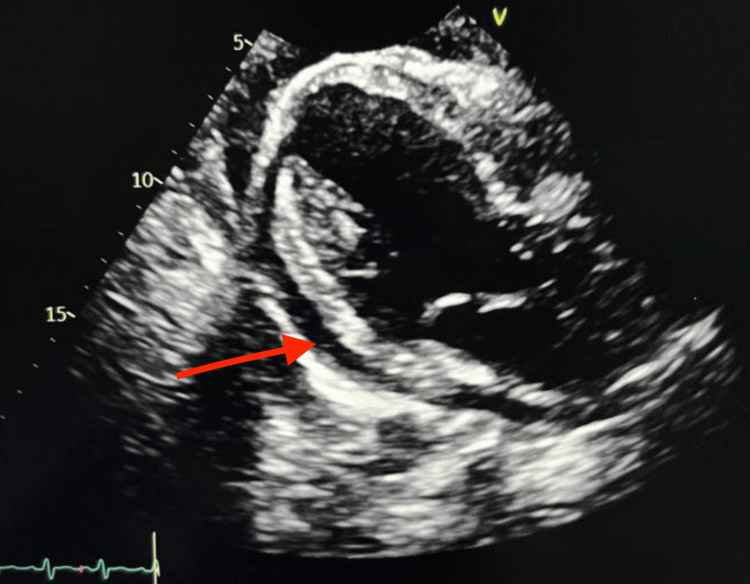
Follow-up echocardiogram taken postoperatively, which demonstrated an ejection fraction (EF) of 20%-25% with trace pericardial effusion and associated hematoma
